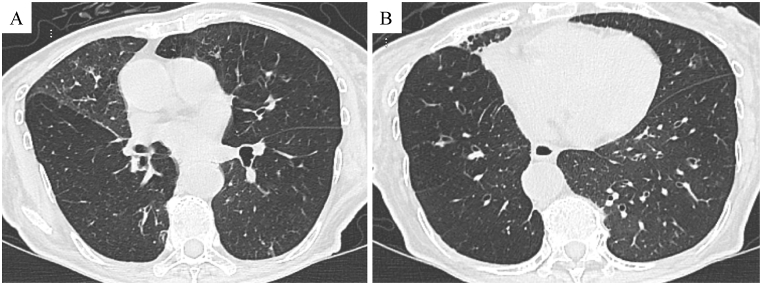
Chest expiratory computed tomography (CT) image shows decreased lung field density in the right lower lobe compared with the right middle lobe (A). Similar to the inspiratory CT results, bronchial dilatation was observed in the lower lobes of both lungs (B).
